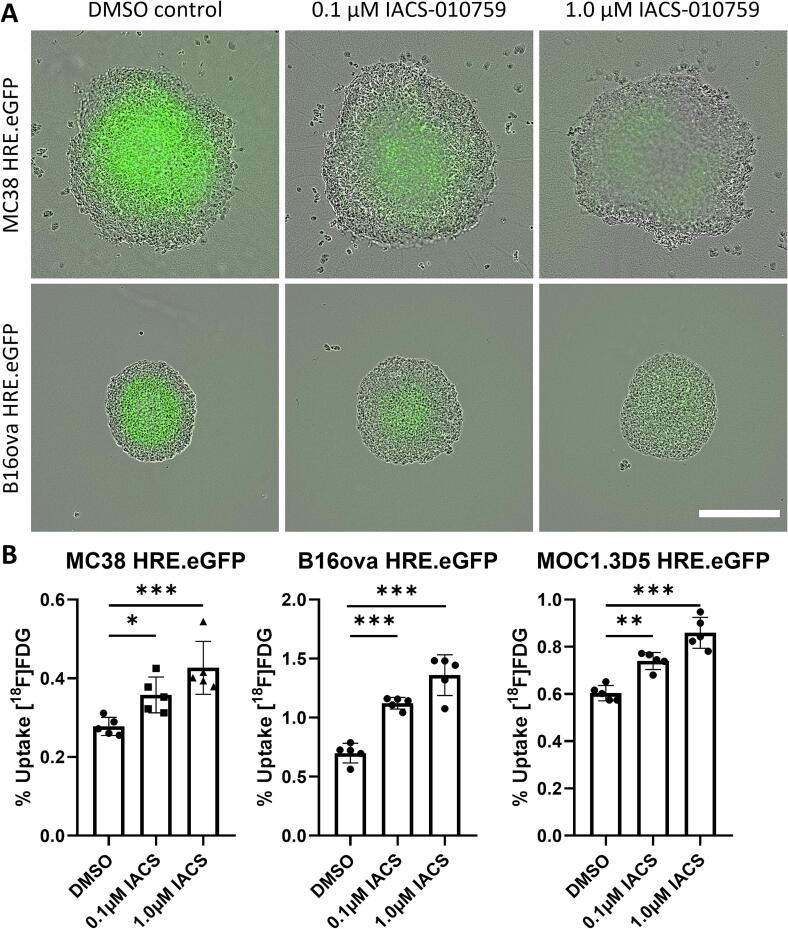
OXPHOS inhibition reduces hypoxia and promotes glucose uptake in tumor spheroids. (A) Fluorescent images of MC38 HRE.eGFP and B16ova HRE.eGFP spheroids 3 h post treatment with IACS-010759 or DMSO. Scalebar represents 500 μm. (B) Uptake of [^18^F]FDG in MC38 HRE.eGFP, B16ova HRE.eGFP and MOC1.3D5 HRE.eGFP spheroids 3 h post treatment with IACS-010758 or DMSO.
